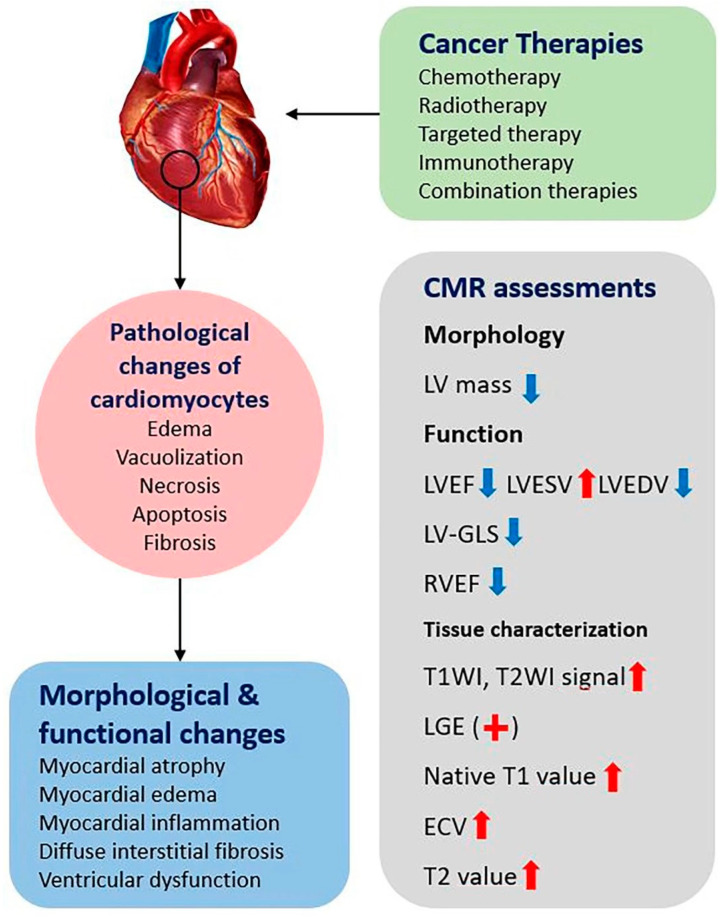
Overview of the pathological changes of cardiomyocytes in cardiotoxicity induced by cancer therapies, the resulting morphological and functional changes of the heart, and CMR assessments that have been performed in previous studies. Different CMR parameters may increase (red arrows) or decrease (blue arrows) or be positive (red plus) in cardiotoxicity. LV = left ventricular; LVEF = left ventricular ejection fraction; LVESV = LV end-systolic volume; LVEDV = LV end-diastolic volume; GLS = global longitudinal strain; RVEF = right ventricular ejection fraction; T1WI = T1-weighted imaging; T2WI = T2-weighted imaging; LGE = late gadolinium enhancement; ECV = extracellular volume fraction.
